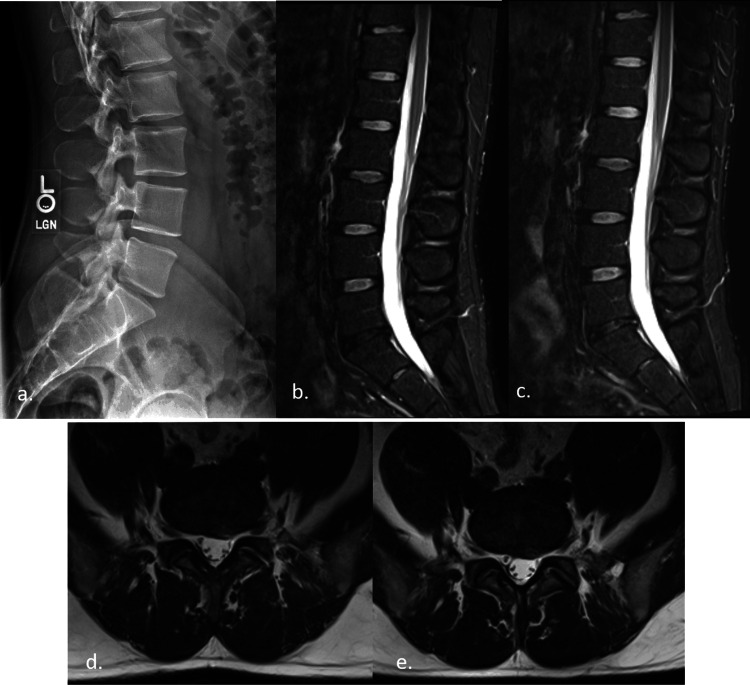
Baseline imaging shows changes in the L5/S1 IVD space and disc conditions, with no significant post-treatment improvement. Baseline lateral lumbar radiographs showed a minimal decrease in the L5/S1 IVD space (a). Baseline sagittal STIR sequence demonstrates Pfirrmann grade 3 at L5/S1 (b) without qualifiable interval change in the post-treatment image (c). Baseline axial T2-weighted sequence shows a disc protrusion with high-intensity zone mildly effacing the thecal sac and left S1 nerve root (d) without qualifiable interval change post-treatment (e). STIR: short tau inversion recovery; IVD: intervertebral disc
